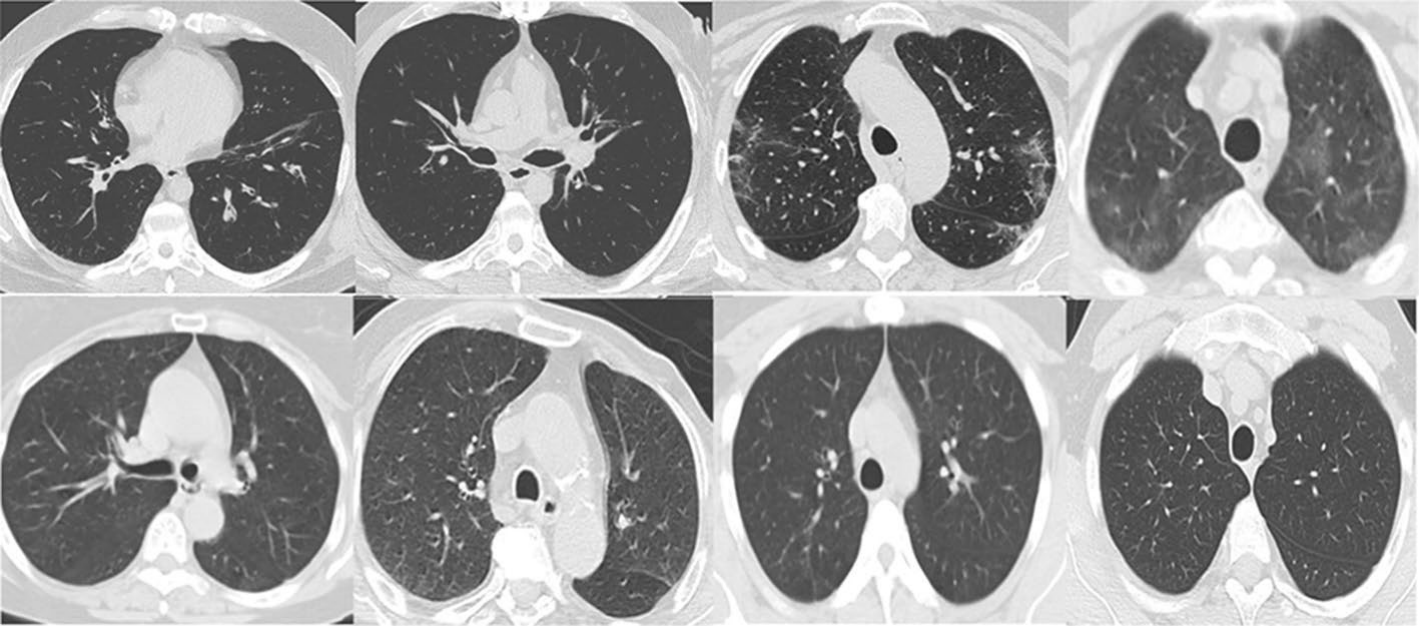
Sample CT slices of COVID-19 images (top row) and Non-COVID images (bottom row).
